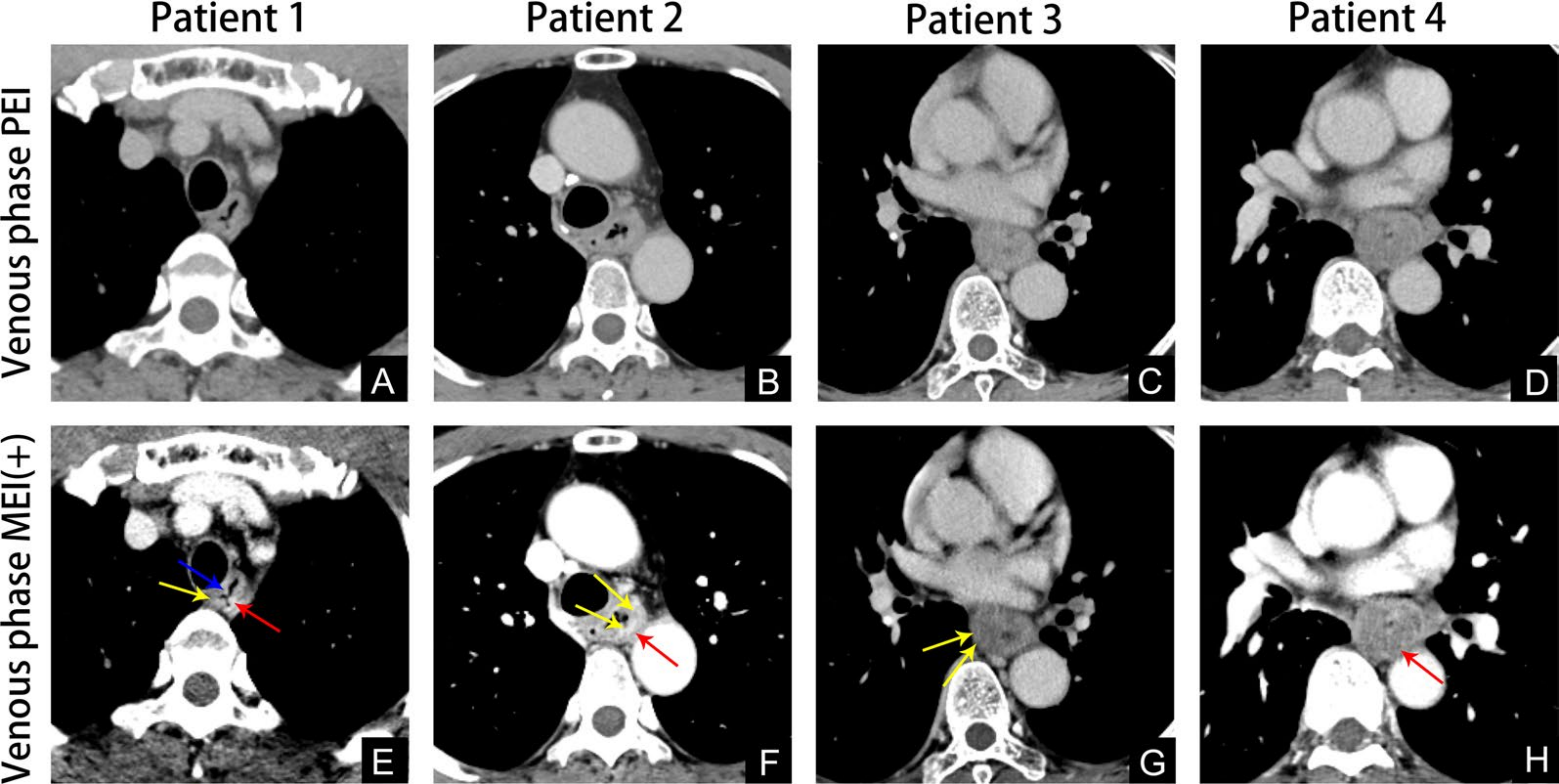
A and E, 66-year-old man with esophageal cancer, venous phase PEI image A, venous phase MEI (+) 40 keV image E, PEI image CT cannot distinguish the tumor, MEI (+) 40 keV shows the high-density muscularis mucosae (blue arrow) and muscularis propria (yellow arrow) are interrupted, and the lesion (Red arrow) is staged as T1b. B and F, 64-year-old man with esophageal cancer, venous phase PEI image B, venous phase MEI (+) 40 keV image F, PEI image CT cannot distinguish the boundary of tumor, MEI (+) 40 keV shows the tumor-invaded muscularis propria (yellow arrow) and the boundary of lesion is clear (Red arrow). The lesion is staged as T2. C and G, 69-year-old man with esophageal cancer, venous phase PEI image C, venous phase MEI (+) 40 keV image G, PEI image CT show the boundary of tumor is not clear, MEI (+) 40 keV shows the tumor-invaded serosa (yellow arrow) and cystic necrosis was evident in the lesion. The lesion is staged as T3. D and H, 62-year-old man with esophageal cancer, venous phase PEI image D, venous phase MEI (+) 40 keV image H, PEI and MEI (+) 40 keV image CT all show the tumor-invaded descending aorta (red arrow). The lesion is staged as T4b
